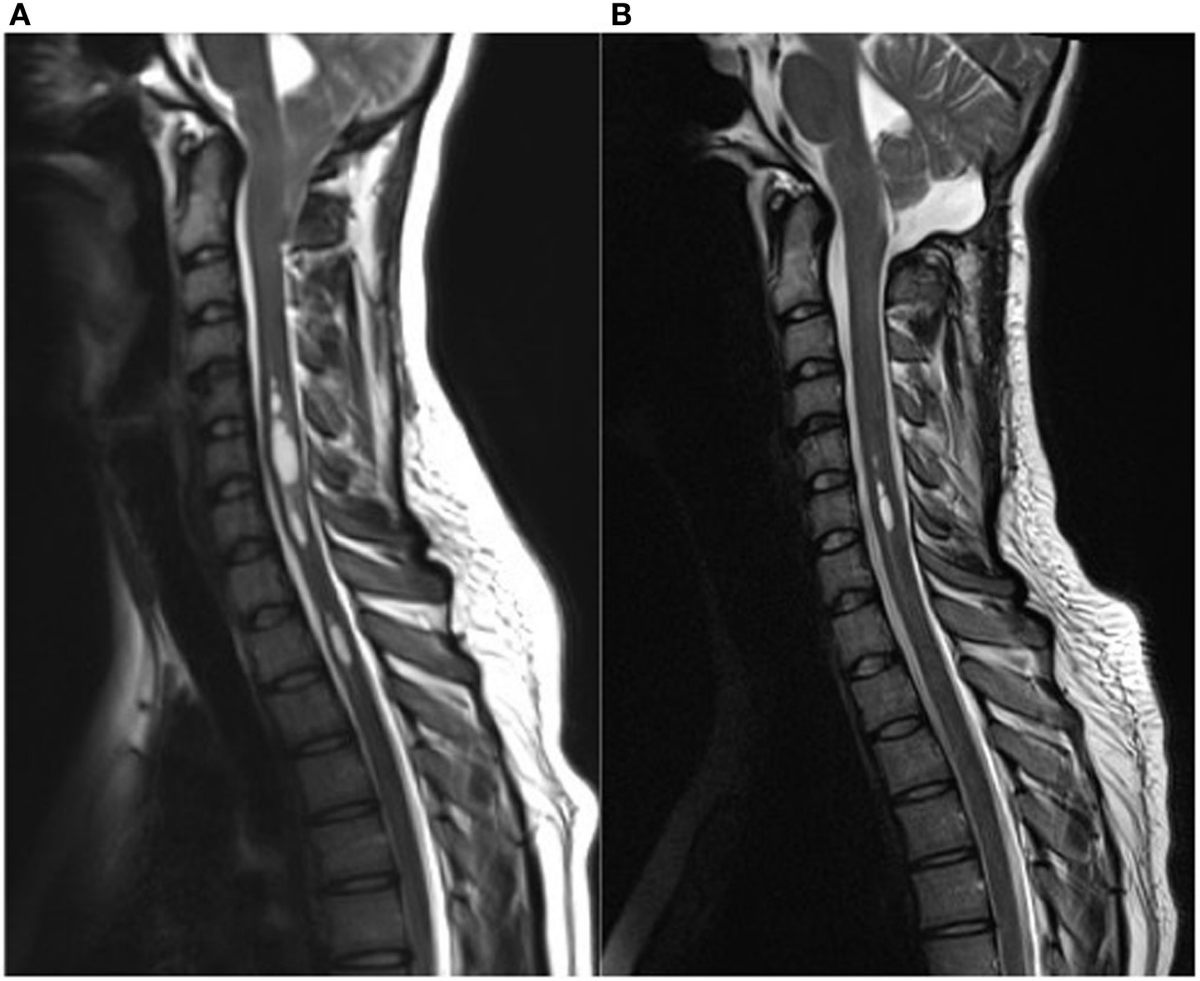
Syringomyelia due to Chiari type I malformation pre (A) and 3 months post (B) surgical decompression. (A) This 19-year-old female complained about occipital headache, hypesthesia of the upper limbs, as well as vertigo attacks. Preoperative sagittal T2-weighted MR images demonstrate tonsil descent down to the lower margin of the arch of C2 and a significant multi-compartmental syringomyelia in the cervical spinal cord. (B) Postoperative MR images clearly highlight a marked reduction of preexisting syrinx as well a reduced tonsillar length at the foramen magnum. The patient reported a remarkable improvement of the symptoms after surgical decompression.
